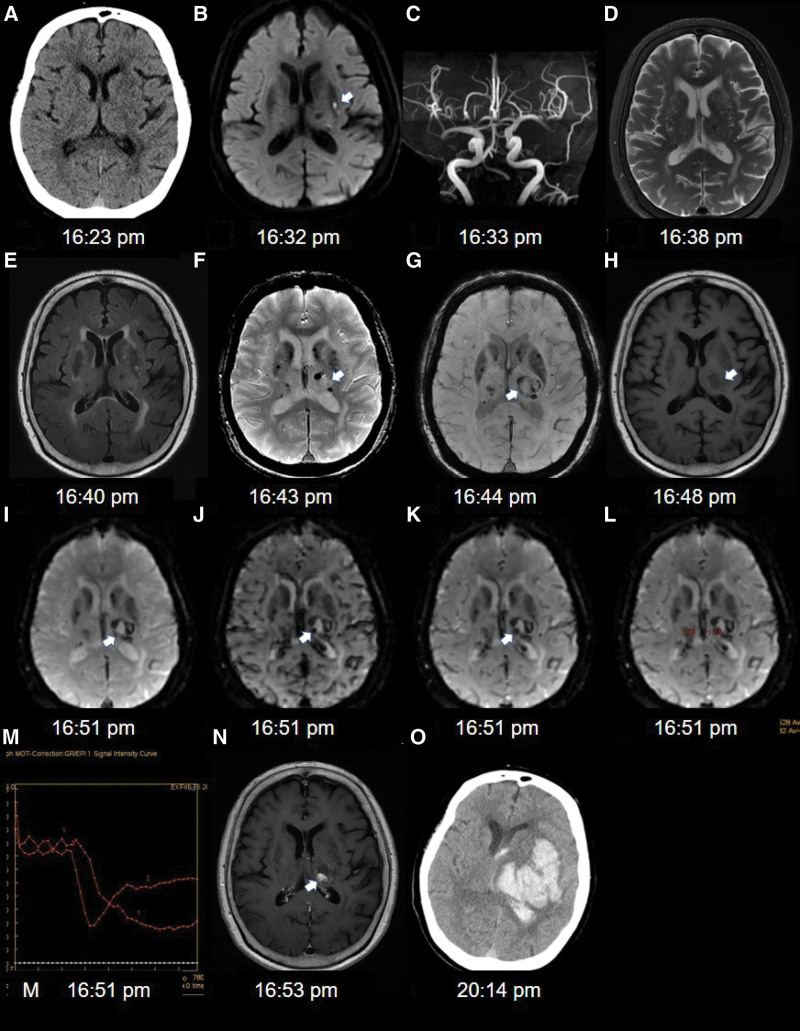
An 88-year-old female patient presented with acute mild dysarthria. (A) Non-contrast head CT shows no evidence of intracerebral hemorrhage, (B) DWI shows acute infarct in the left basal ganglia (arrow), (C) MRA shows a normal appearance of the intracranial vessels, (D and E) T2WI and FLAIR image show no definite hyperintensity in the left thalamus, (F) GRE image shows multiple microbleeds in both the basal ganglia and thalamus. Mild hyperintense signal change is observed in the left thalamus, which is absent in previous T2WI and FLAIR image (arrow), (G) SWI shows a dark signal rim around the periphery of the hyperacute hematoma in the left thalamus (arrow), (H) T1WI demonstrates a lesion of low signal intensity in the same area as GRE image (arrow), (I–K) In T2*-weighted images from DSC-MRI, an area of dark signal intensity disperses and spreads out within the hematoma (arrow). The time interval between images is 20 seconds, (L and M) In the region of interest (ROI 1), the time-intensity curve shows a persistent signal decrease compared with that in the contralateral normal region of interest (ROI 2), (N): Post-contrast T1WI shows a collection of extravasated contrast along the margin of the hematoma (arrow), and (O) Follow up non-contrast head CT at 3 hours after the MRI demonstrates marked enlargement of the hemorrhage. CT = computed tomography, DSC = dynamic susceptibility contrast, DWI = diffusion-weighted imaging, FLAIR = fluid-attenuated inversion recovery, GRE = gradient recall echo, MRA = magnetic resonance angiography, MRI = magnetic resonance imaging, SWI = susceptibility-weighted image, T1WI = T1-weighted imaging, T2WI = T2-weighted imaging.
